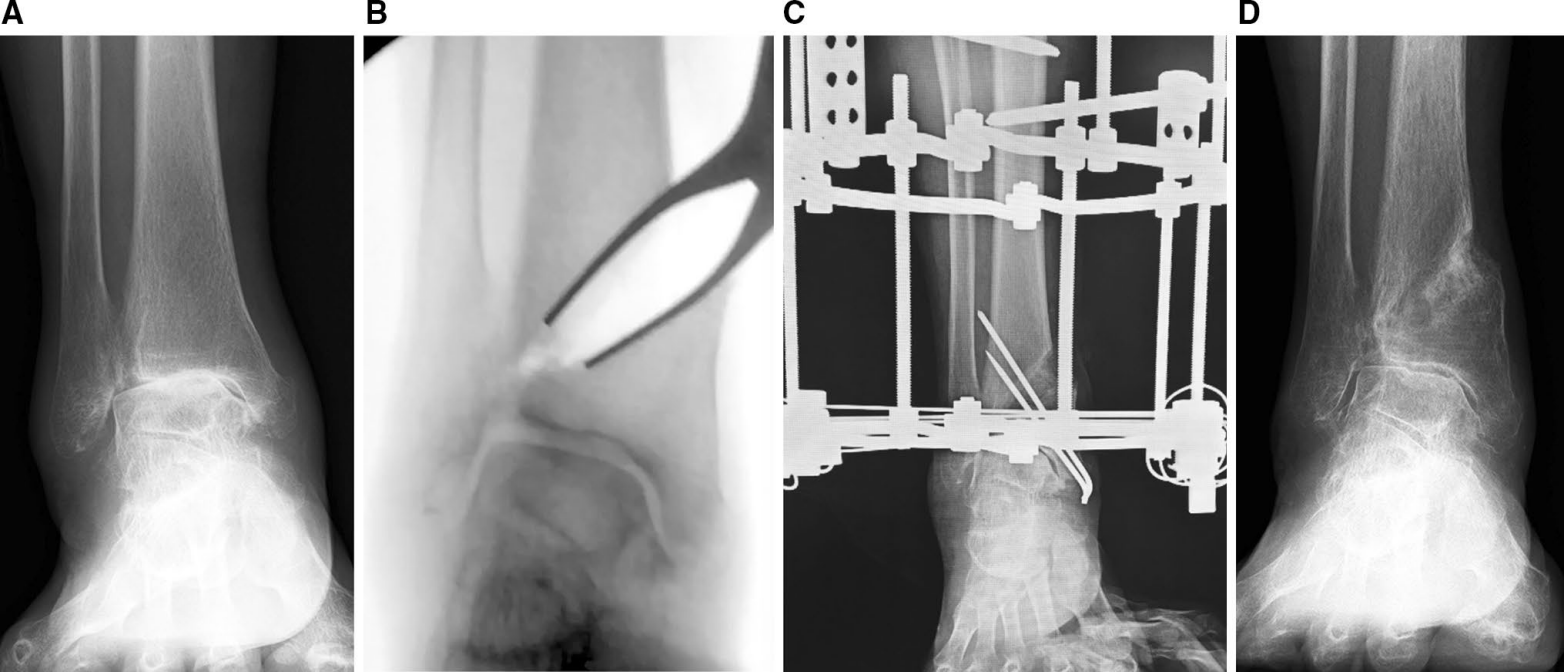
A 71-year-old woman with stage 3b varus ankle osteoarthritis of her right ankle underwent a DTOO. The weight-bearing radiograph before DTOO showed joint space narrowing and even bone-to-bone contact over the dome of the talus (a). The intra-operative radiograph shows separation of the DTOO by laminar spreader (b). The separation was continued until the articular surface of the lateral talar body came into contact with the medial articular surface of the lateral malleolus. Osteotomy fixation was with the Ilizarov external fixator and supplementary Kirschner wires (c). Weight-bearing radiographs 2 years after DTOO shows good restoration of ankle joint and with the plantar surface of the hindfoot perpendicular to the tibial axis (d). Clinical review confirmed complete resolution of ankle joint pain. The range of motion was decreased slightly after DTOO (dorsiflexion 0°—plantar flexion 15°), but the patient was able to return to farm work
